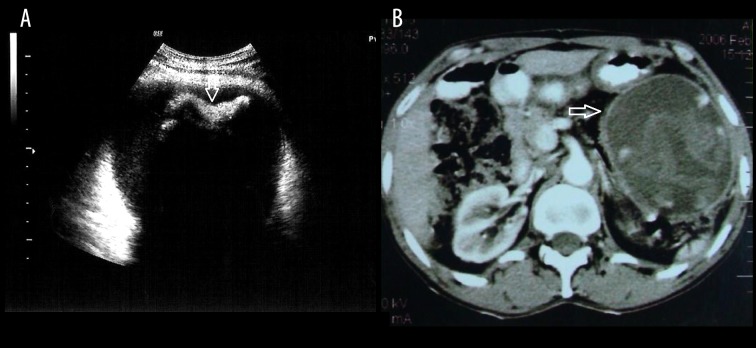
(A) Sonogram reveals a cystic lesion containing a linear, hyperechoic structure demonstrating dense posterior acoustic shadowing. (B) Contrast-enhanced CT scan of the abdomen shows a thin-walled, cystic mass in the left lumbar region with internal linear densities showing a characteristic infolding/whorled configuration.
